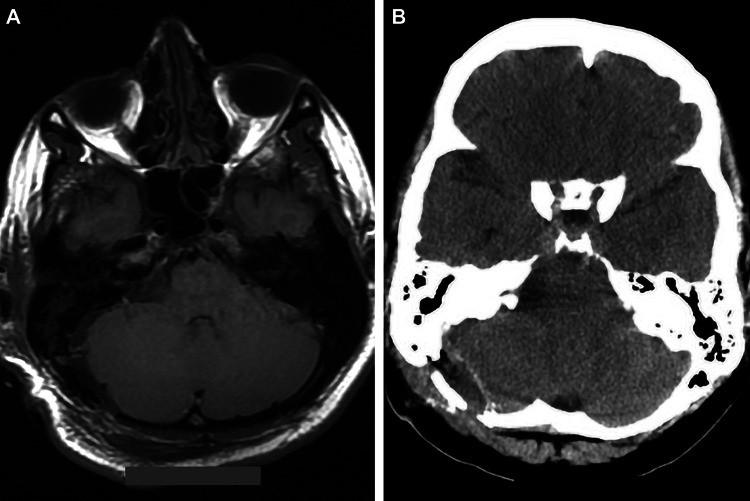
Case 5 – a 42-year-old woman with a known case of hypercoagulopathy who presented with right-sided hearing loss and facial weakness and received a diagnosis of right cerebellopontine (CP) angle mass (A) Postoperative axial T1-weighted MRI showed changes consistent with a retrosigmoid craniotomy for resection of a right cerebellopontine (CP) angle mass. (B) Axial CT from postoperative day nine, performed because of a syncopal episode, demonstrated stable postoperative changes and a stable amount of extra-axial fluid and gas deep in the craniotomy site. There was no evidence of a superimposed acute intracranial process MRI: magnetic resonance imaging; CT: computed tomography Used with permission from Barrow Neurological Institute, Phoenix, Arizona
